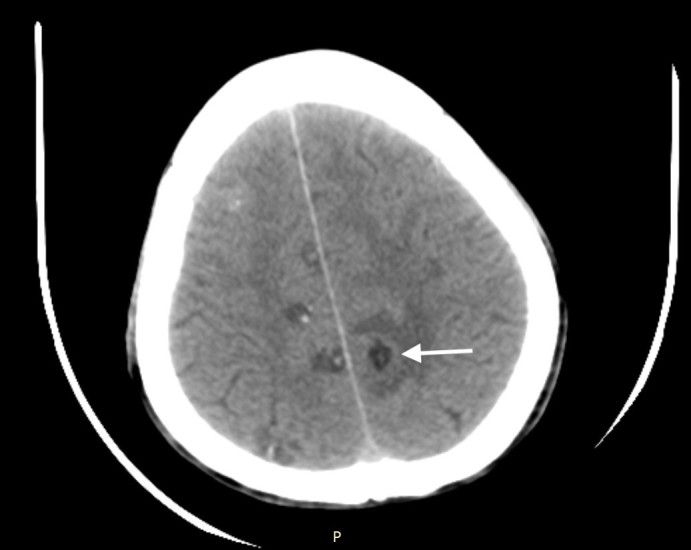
Computed tomography of the brain showing multiple cystic lesions in vesicular and colloid-vesicular stage with lesion in left cerebral hemisphere demonstrating surrounding edema (arrow).
